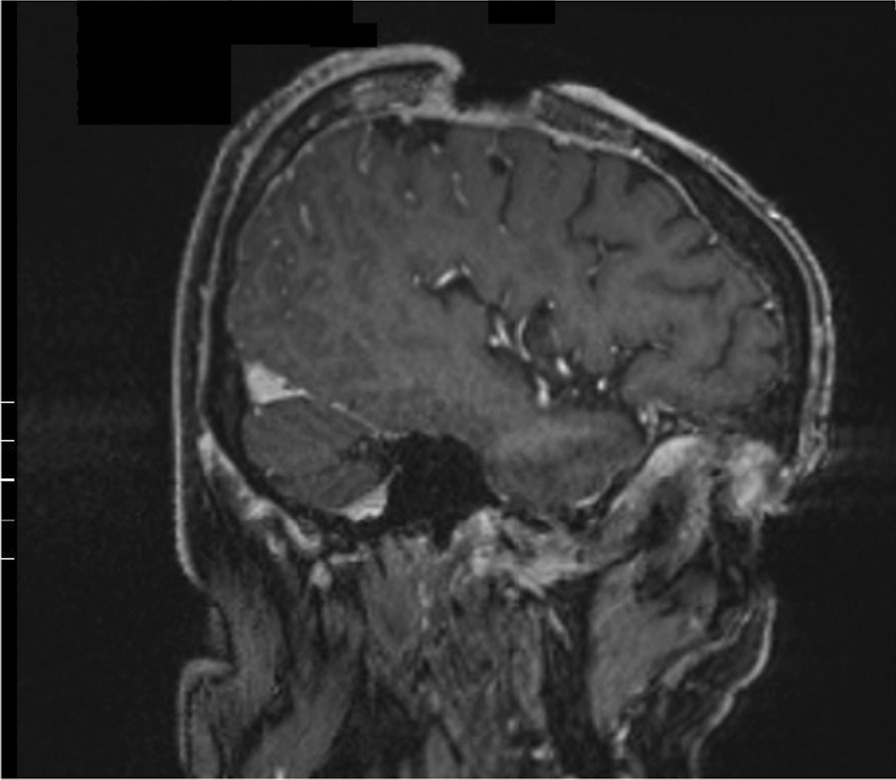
T1 MP-RAGE 3D weighted sagittal contrast-enhanced MRI sequence 3 months after radiotherapy. MR image showed a dramatic response to definitive radiotherapy with residual inflammatory tissue on the base of the initial ulcer.
